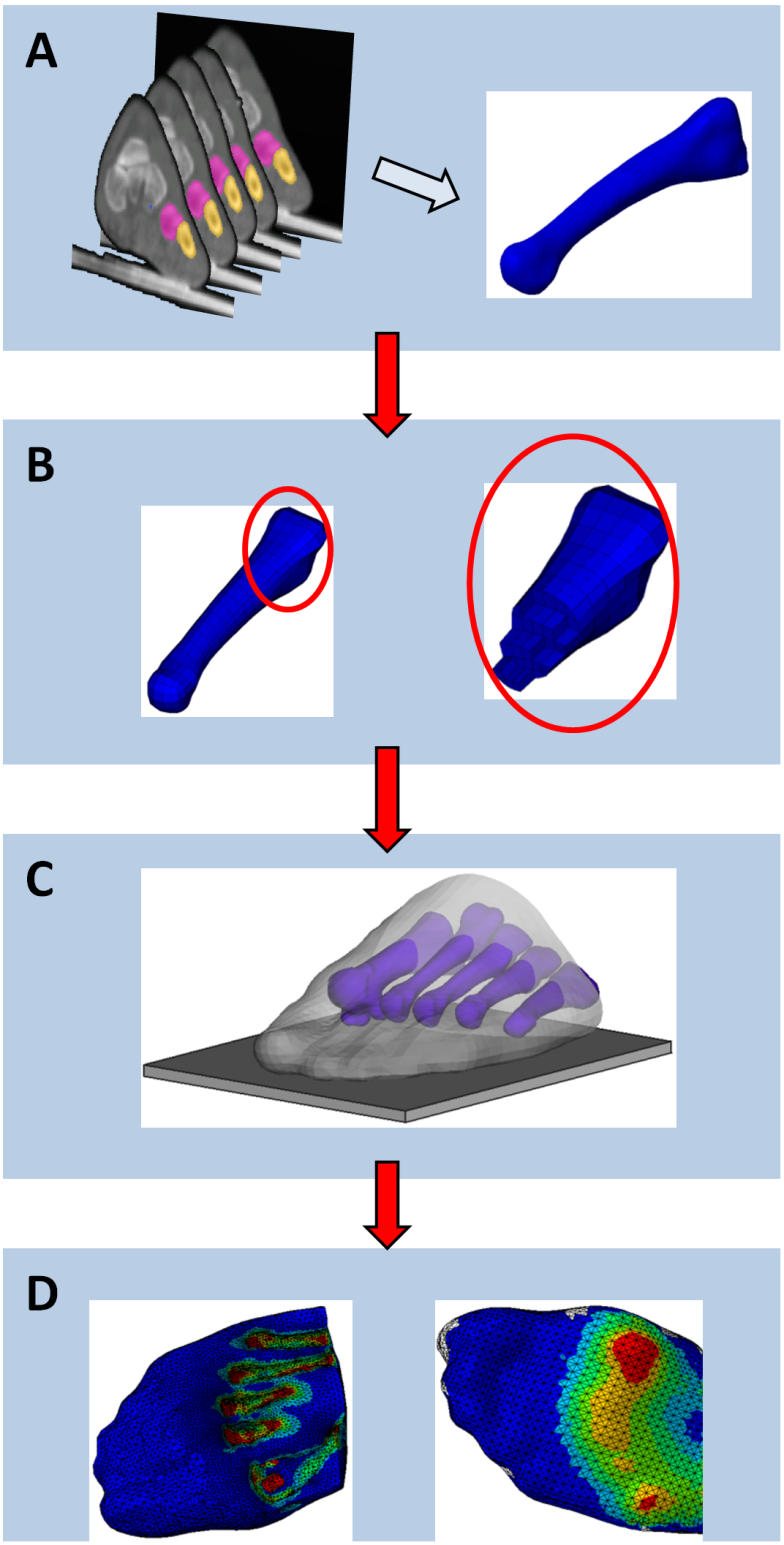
Development process for FE model of the foot. (A): Geometry of part constructed from CT slices of foot; (B): parts meshed into elements; (C): parts combined and interactions between them defined; (D): results of analysis, von Mises stresses (left) and plantar contact pressures (right).
